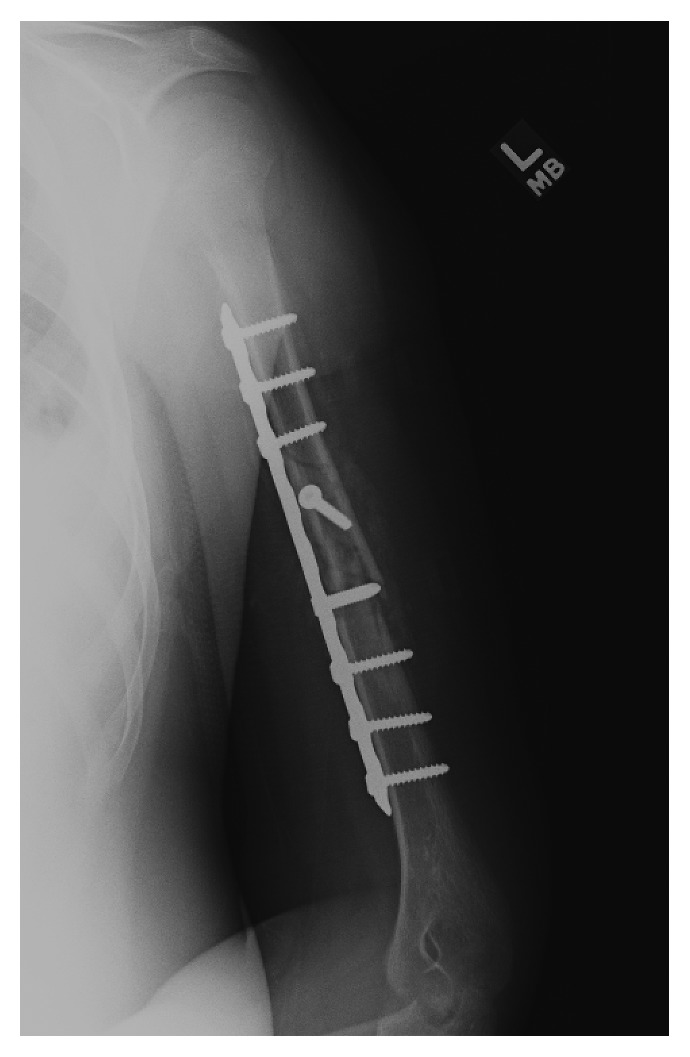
Anteroposterior radiograph following open reduction internal fixation with intact hardware and the fracture in anatomic alignment.
